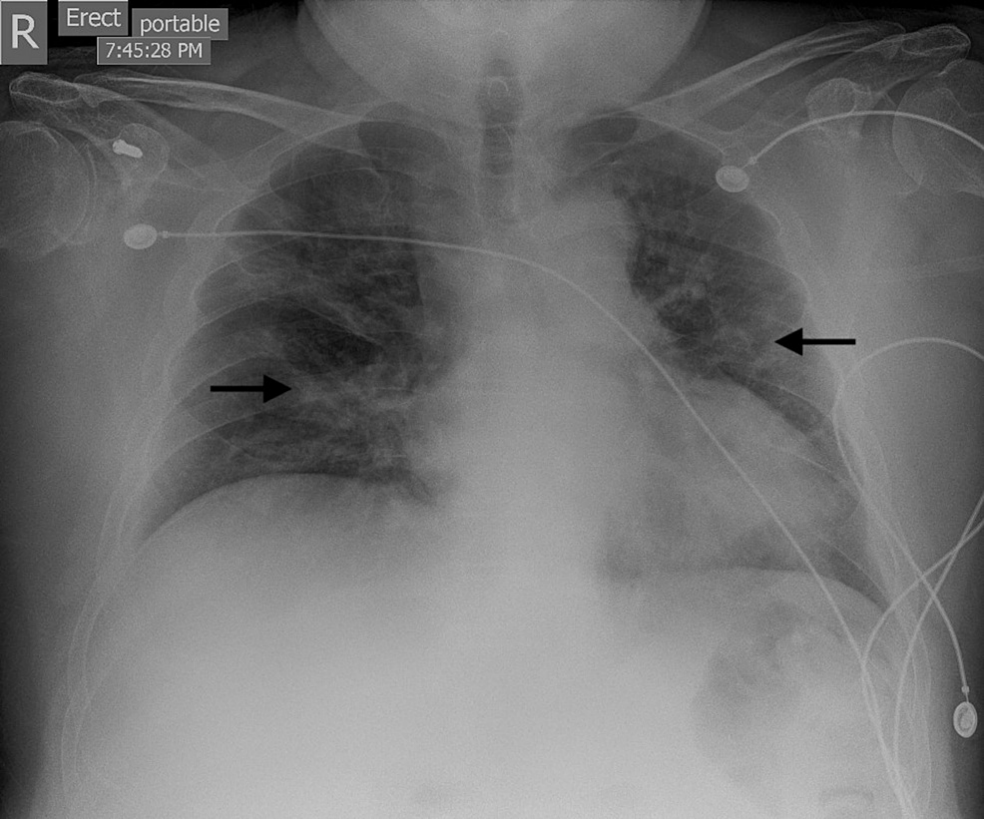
Admission chest x-ray demonstrating bilateral pulmonary infiltrates (arrows).
